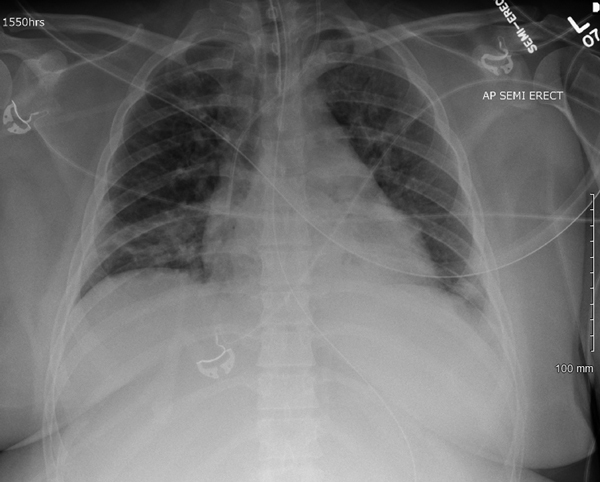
Chest radiograph obtained (with portable machine) of semirecumbent adult patient with enterovirus D68–associated acute respiratory distress syndrome on hospital day 3.
